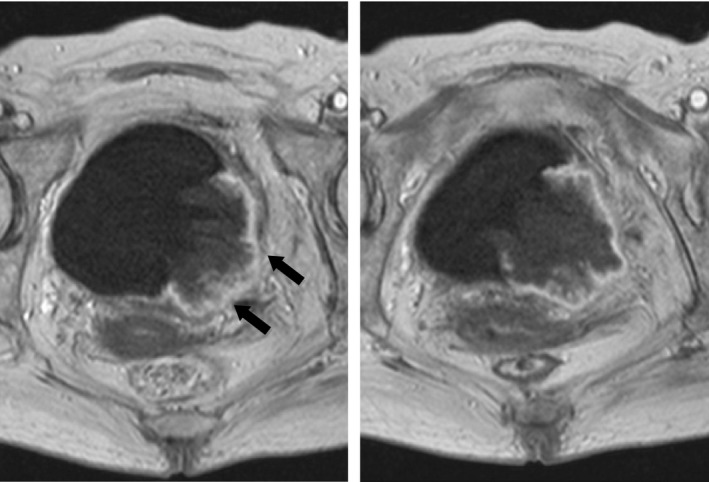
Contrast‐enhanced magnetic resonance imaging of the bladder revealed the interruption of muscle layer, and the depth of invasion was diagnosed as T2.
